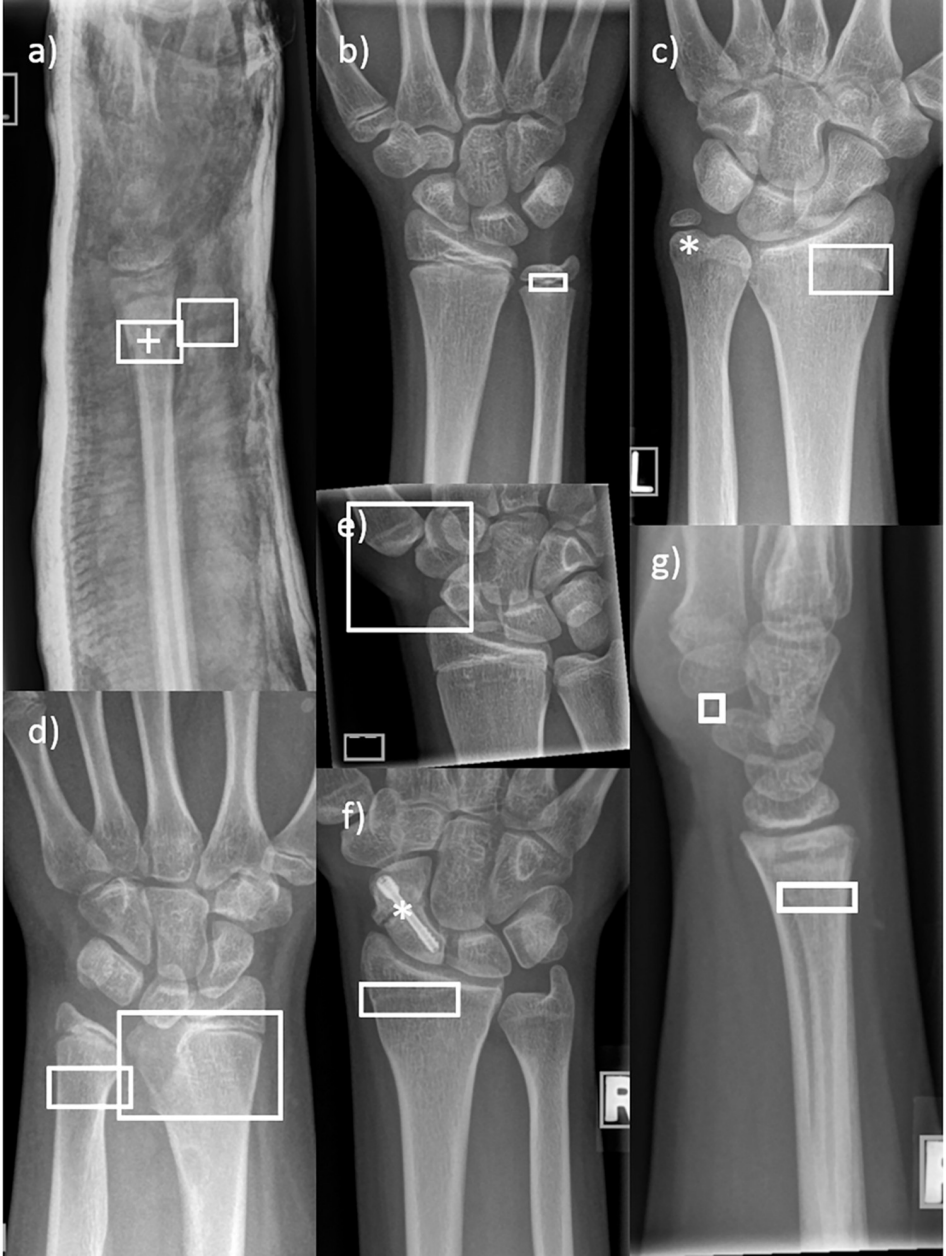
Different cases of erroneously annotated fractures in pediatric wrist radiographs. a) The cast was mistaken for a fracture. The + sign indicates the second, correctly labeled bone injury. b, c & f) Students marked the ulnar and radial growth plates as a fracture. d) A Madelung’s deformity was mimicking a fracture. e) The carpal bones were mistaken for an injury. g) A so-called Harris line thought to be a fracture. * The stars indicate missed injuries.
